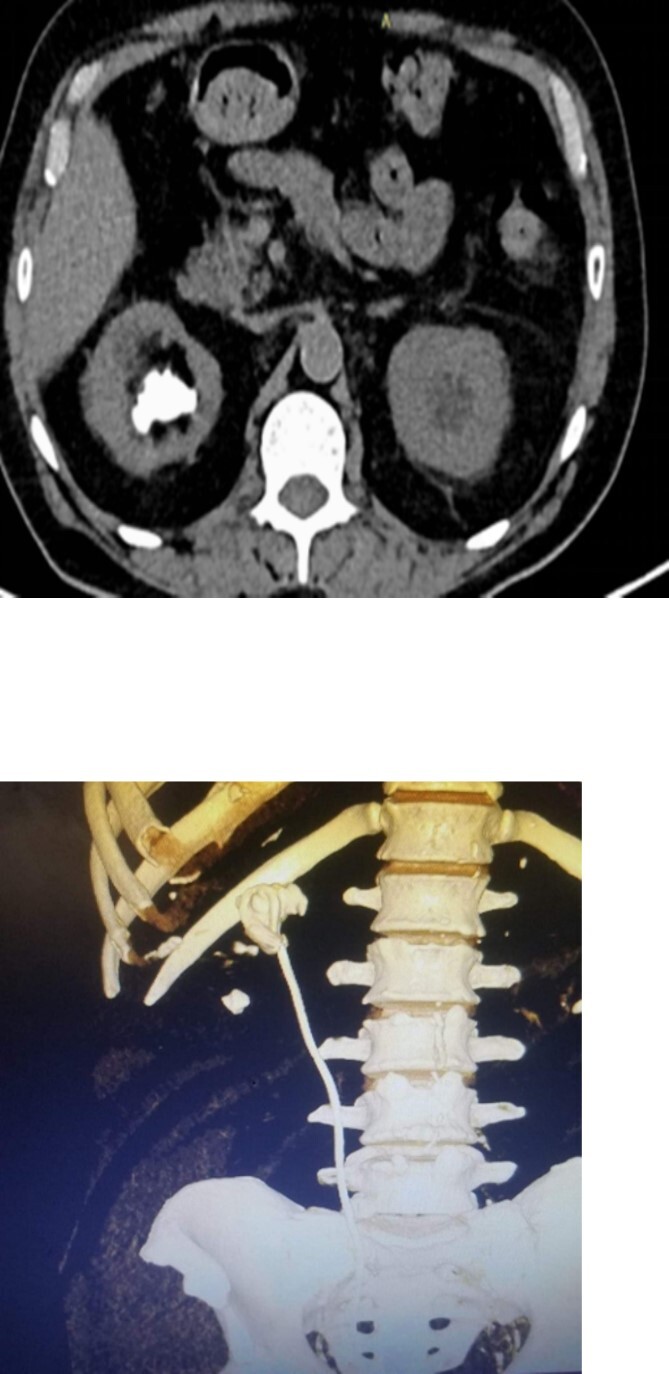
Non-contrast CT scan showed intra-renal stone with significant proximal pigtail calcification and mild degree of hydronephrosis.
